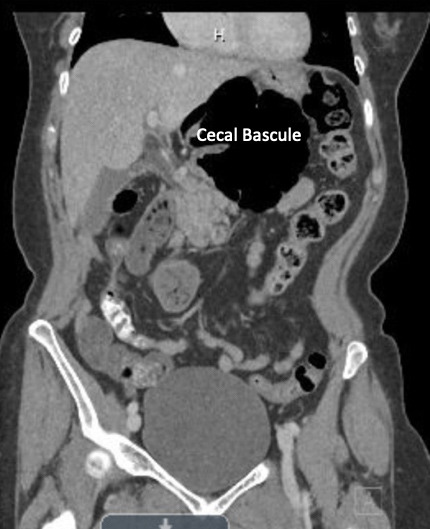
Computed tomographic scan of the abdomen and pelvis revealing cecal bascule herniation through the Foramen of Winslow.
